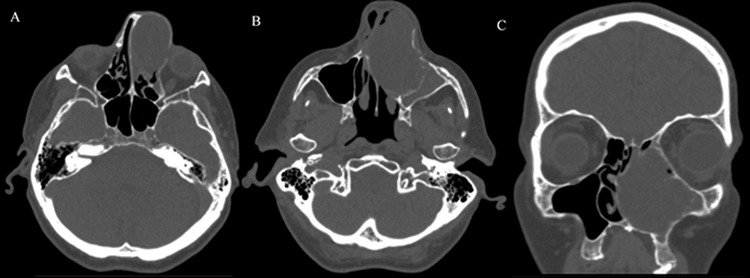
Pre-surgical computed tomography of an inferior turbinate pleomorphic adenoma and secondary maxillary sinusitis. A and B: axial view, C: coronal view
